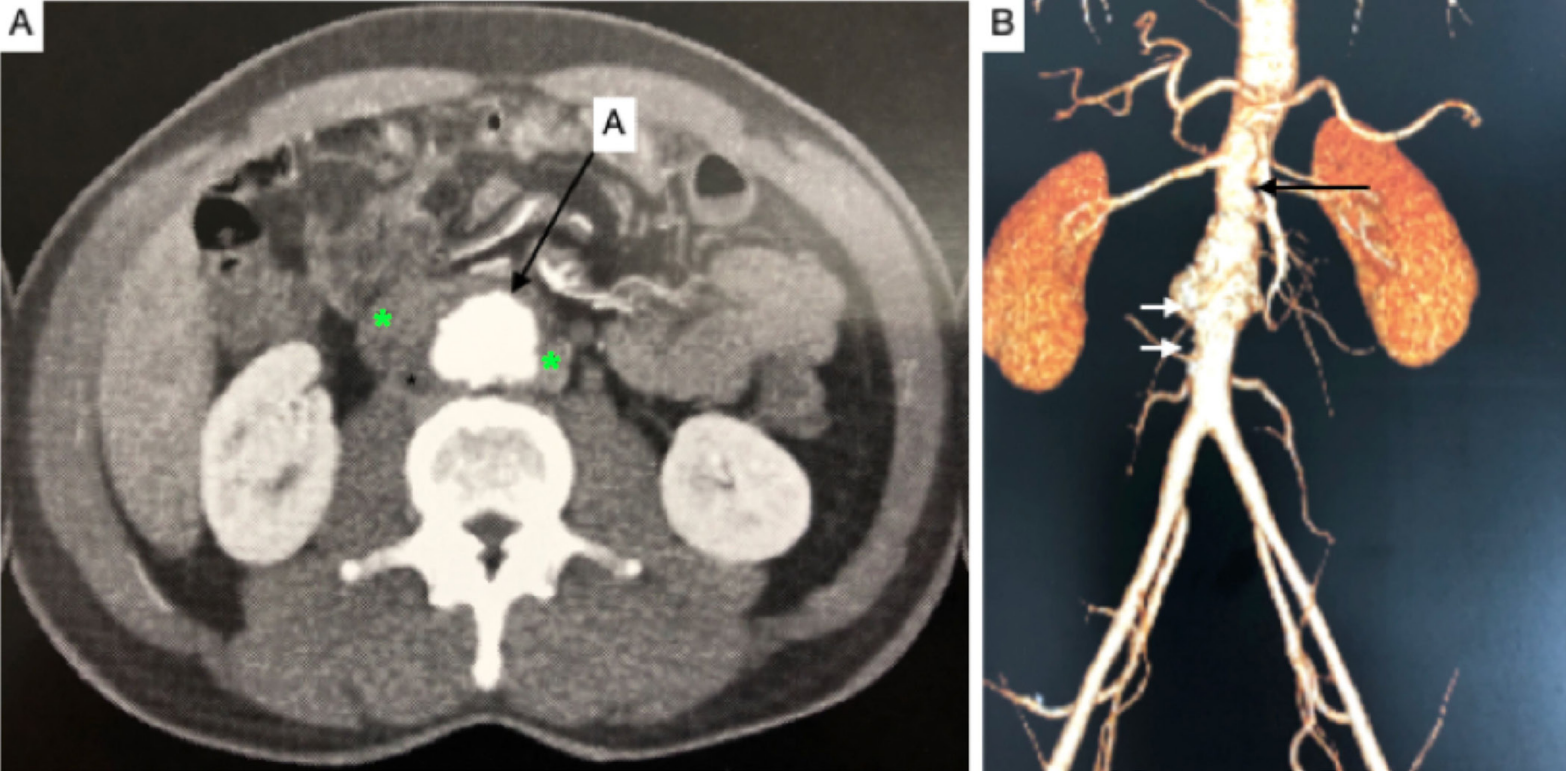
Computed tomography with intravenous contrast. (A) Axial slice; A: aorta; the asterisks mark an image compatible with periaortic collection/mass. (B) Reconstruction with the maximum intensity projection (MIP) technique. Observe the irregular outlines not just of the aneurysm, but also of the aortic segments proximal (black arrow) and distal (white arrows) of the aneurysm.
